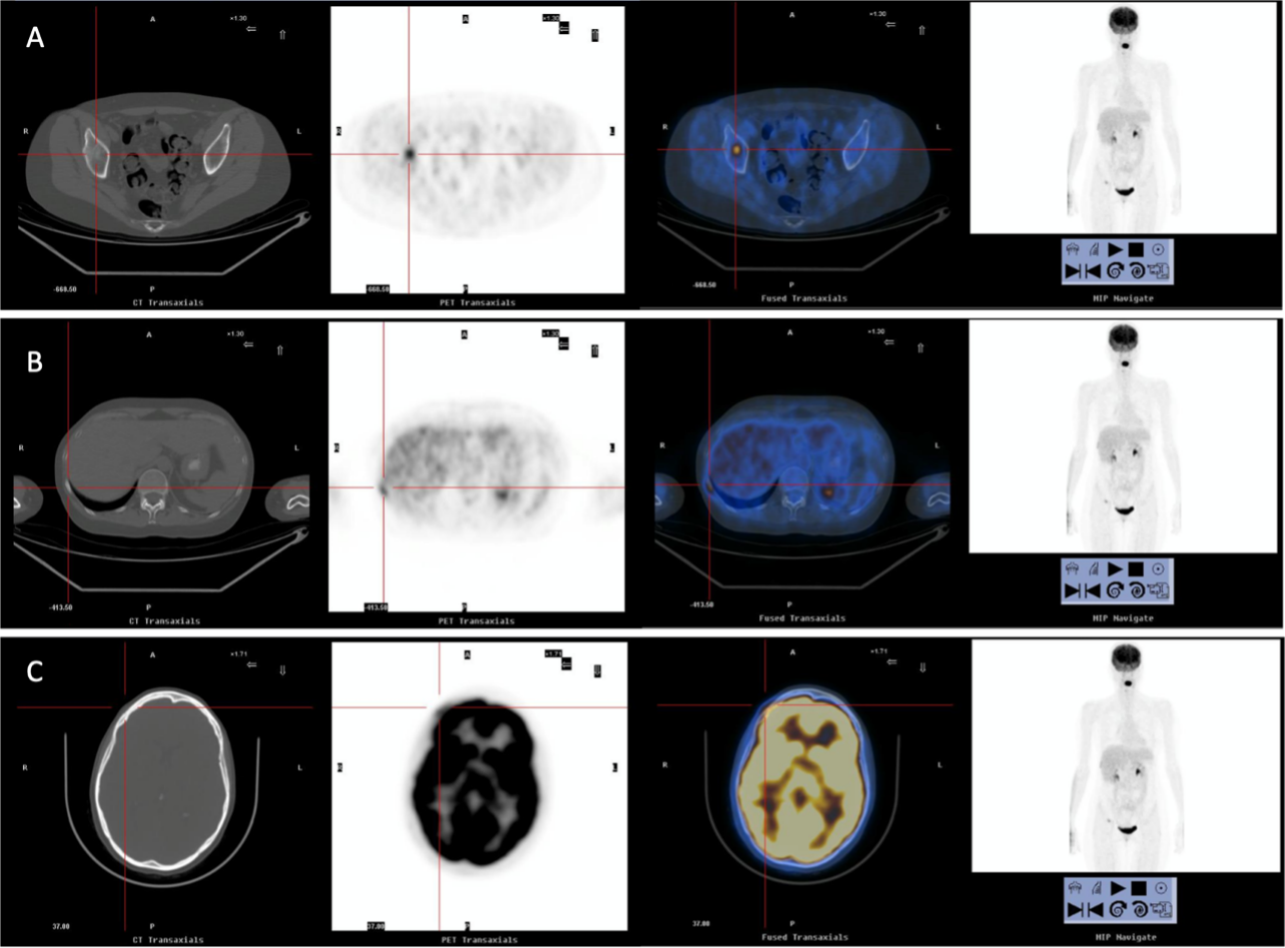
Images from computerized tomography (CT), FDG-PET and bone scintigraphy, showing bone relapse in right acetabulum (A), tenth right rib (B) and right frontal bone (C).
